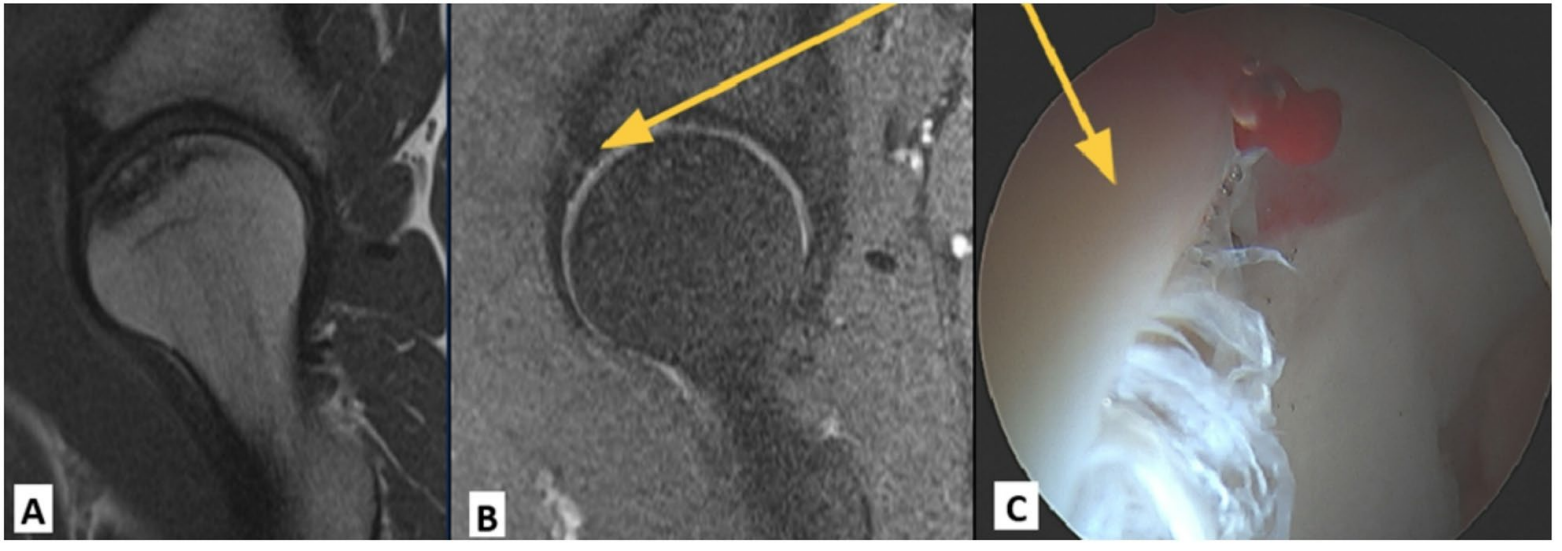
MRI and arthroscopic image of a right hip with Ficat stage 2 avascular necrosis (AVN) of the femoral head. The yellow arrow indicates the location of the labral tear. (A) Sagital T1-weighted MRI with crescentic area of Ficat stage 2 AVN of the femoral head characterized by focal bone marrow edema, sclerotic reactive margin, no evidence of subchondral collapse, and preservation of the joint space. The AVN is localized to geographic zones 2 and 3 of the femoral head. (B) Sagittal T2-weighted MRI with a zone 2 labral tear. (C) Arthroscopic photo viewing form the anterolateral portal redemonstrating the zone 2 labral tear intraoperatively
